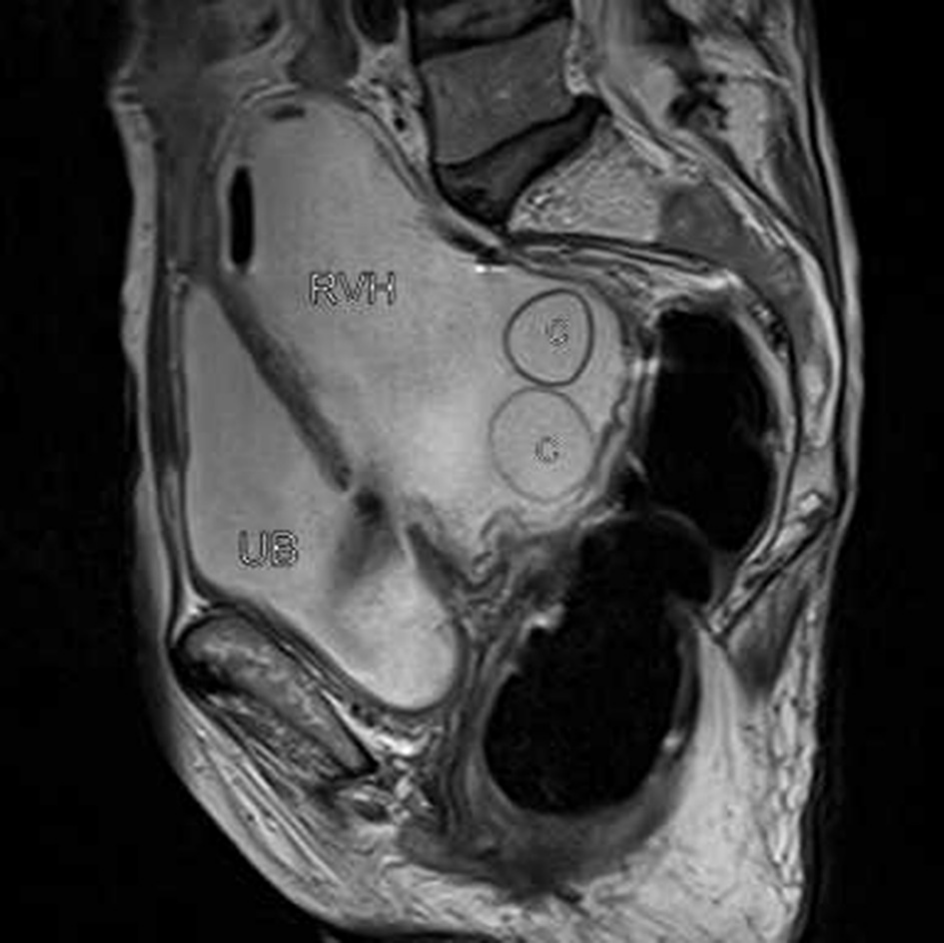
Sagittal *T*_2_ weighted MRI showing a retrovesical cyst (RVH) lying posterior to the urinary bladder (UB) and communicating with it.
